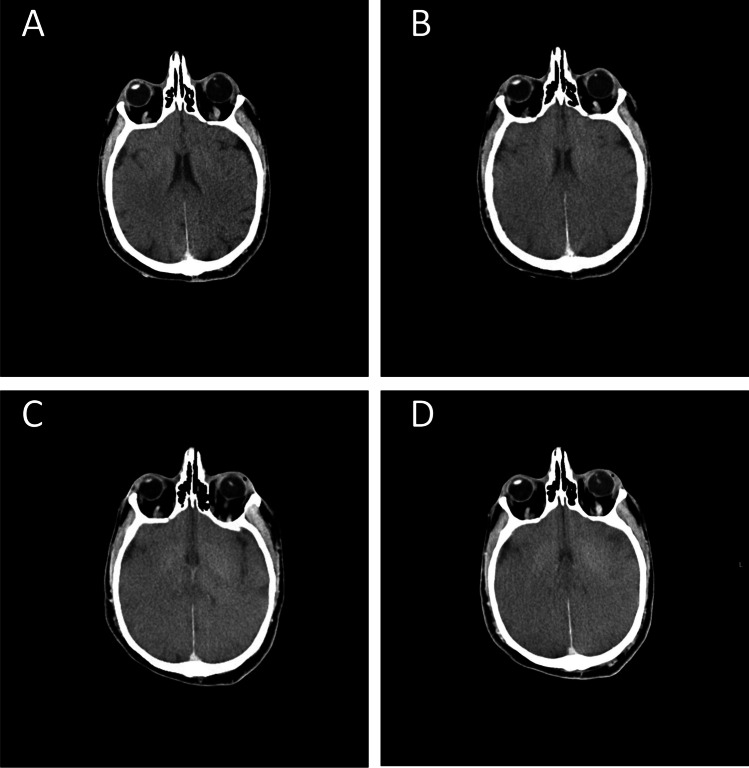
Representative CT brain slices acquired on one of the subjects included in the study at different PMT, namely day 1 (A), day 2 (B), day 3 (C), and day 4 (D)
